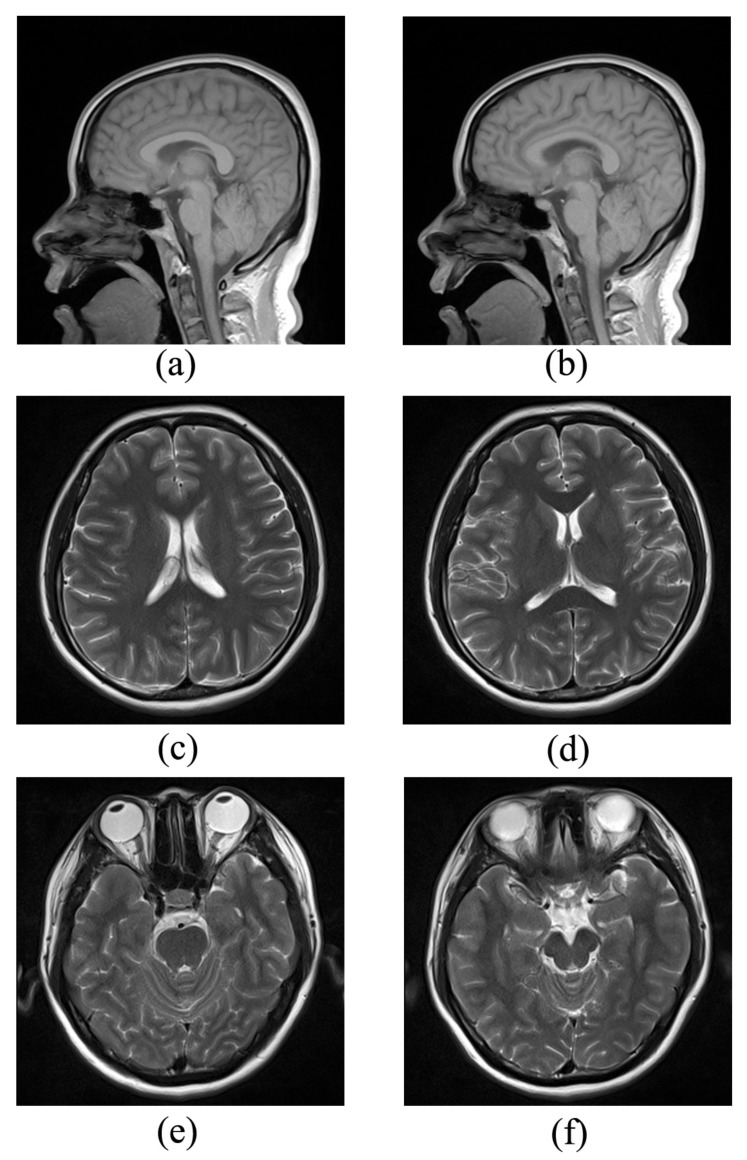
The MR images. Brain A: the reference image (a) and target image (b); Brain B: the reference image (c) and target image (d); Brain C: the reference image (e) and target image (f).
